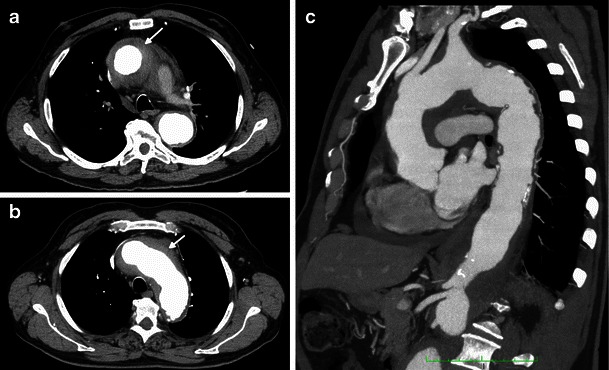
Axial and sagittal enhanced reformatted CT images of the thoracic aorta in a 73-year-old male showing periaortic infiltration (arrows) extending from the aortic root (c) to the descending aorta (a) and circumferentially involving the ascending aorta (a) and aortic arch (b)
